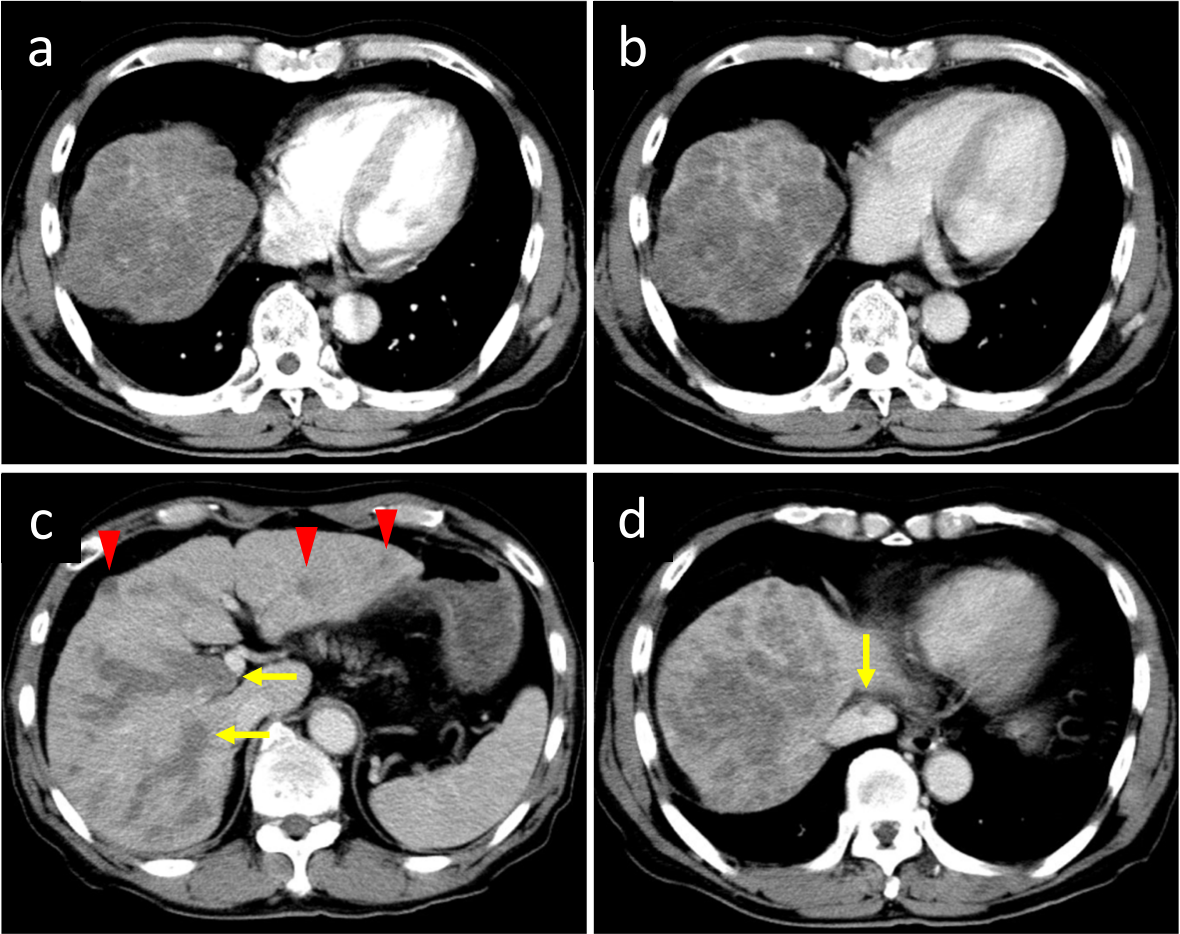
Enhanced CT images before introducing HAIC. Heterogeneous, 13-cm mass in the right liver shows hypovascular appearance in the arterial phase (a) and in the portal phase (b). Massive tumor thrombosis (arrow) growing to the right portal vein (c) and inferior vena cava (d), with bilateral intrahepatic liver metastases (arrowhead) (c). CT, computed tomography; HAIC, hepatic arterial infusion chemotherapy
